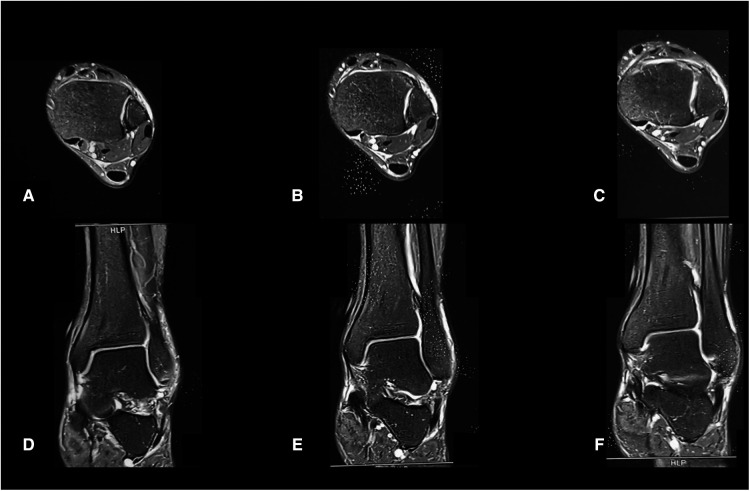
The initial transverse view (A–C) and coronal view (D–F) MRI images of the ankle.
